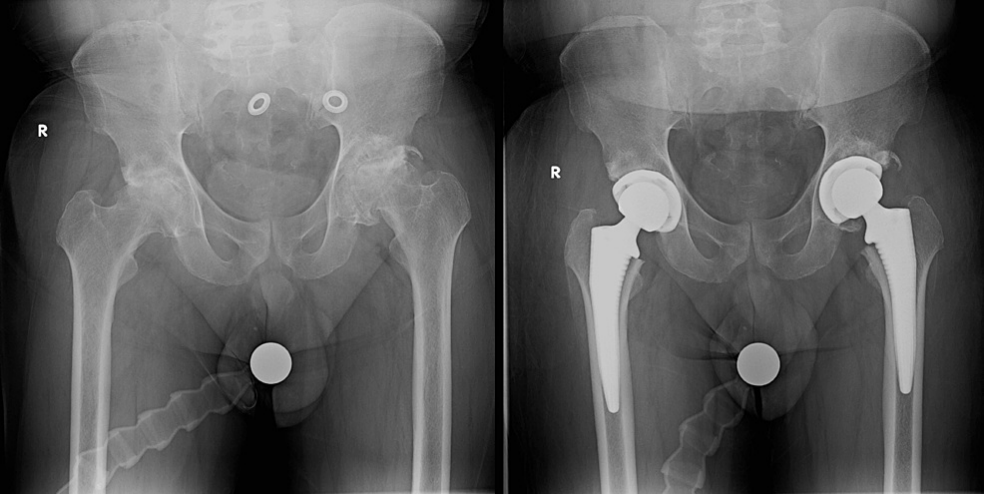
Preoperative (left) and postoperative (right) X-rays of bilateral hip joints
